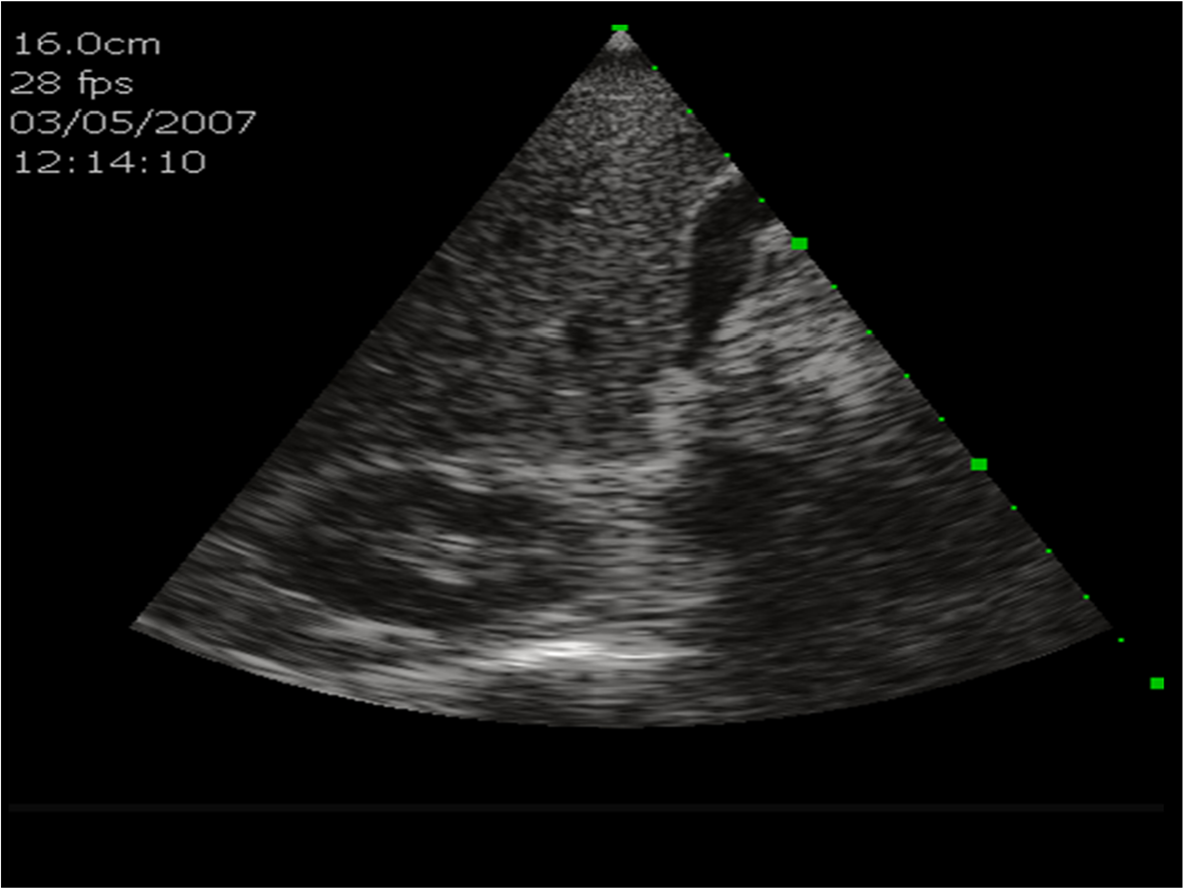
Acuson P10 Siemens: B-scan ultrasound of the liver, including the gall bladder and the right kidney
